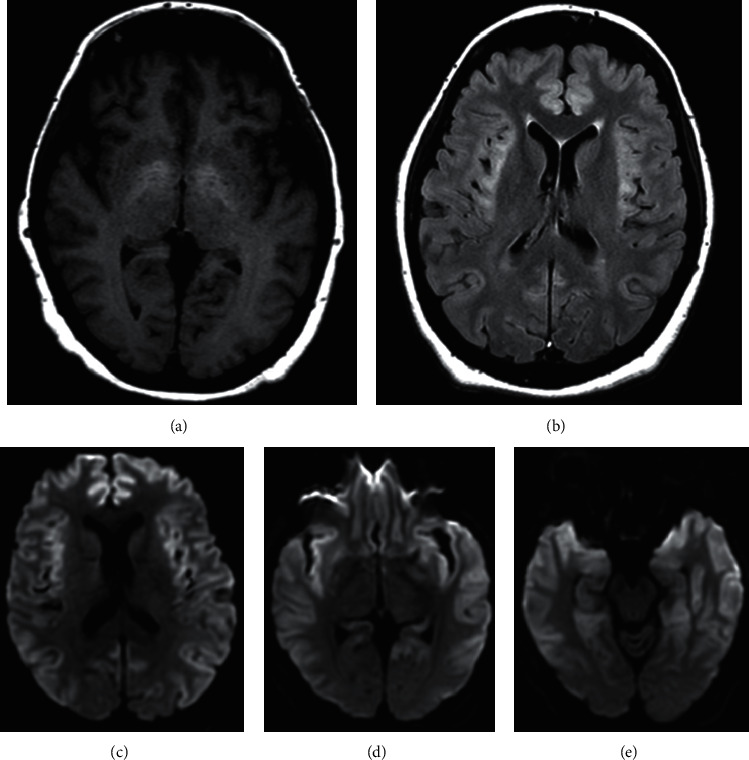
Slices of axial brain MRI performed 15 hours after CT head. (a) T1 sequence showing bilateral hyperintense basal ganglia characteristic of chronic hepatic encephalopathy. (b) T2 FLARE sequence showing bilaterally symmetric intensities of the frontal and insular cortex. (c–e) DWI sequence showing prominent gray matter diffusion restriction of the bilateral frontal, temporal, parietal, and insular cortices.
